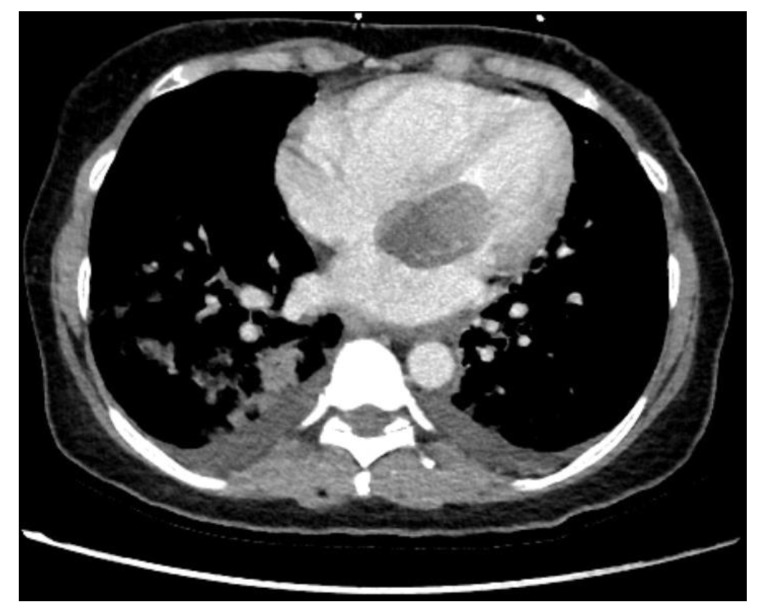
Visualization of a left atrial CM in a computed tomography scan with contrast medium.
